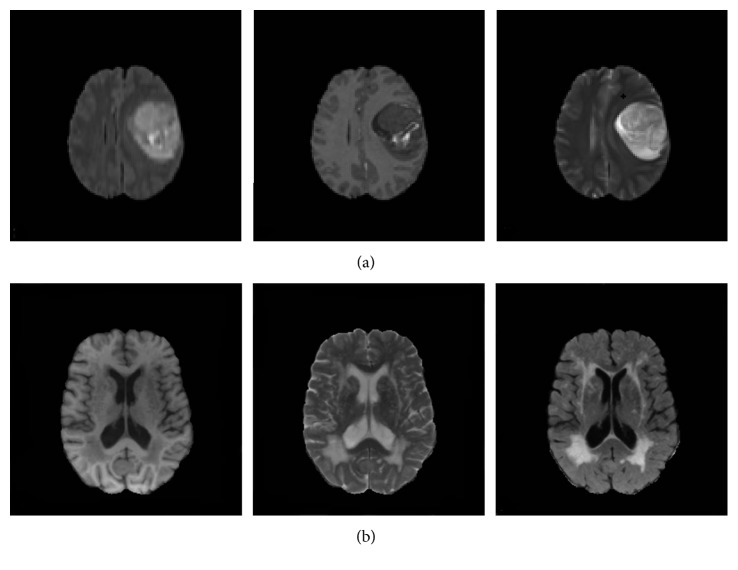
Multisequence MR images of a brain tumor patient (a) and a multiple sclerosis patient (b). (a) Multisequence images of a brain tumor, from left to right: FLAIR, T1-enhancement, and T2 sequences. (b) Multisequence images of multiple sclerosis, from left to right: T1, T2, and FLAIR sequences.
